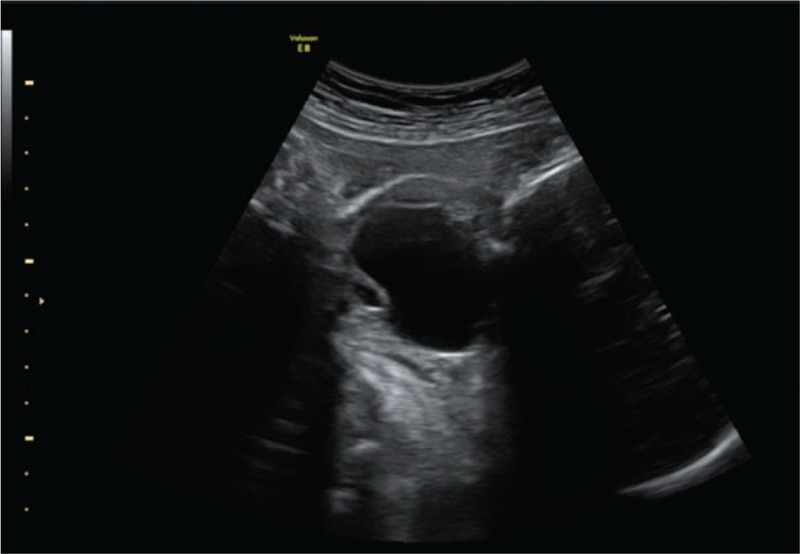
A cystic lesion (size, 4.9 cm × 3.2 cm) is seen in the anterolateral region on the left side of the neck on ultrasound performed at 36 weeks of gestation.
